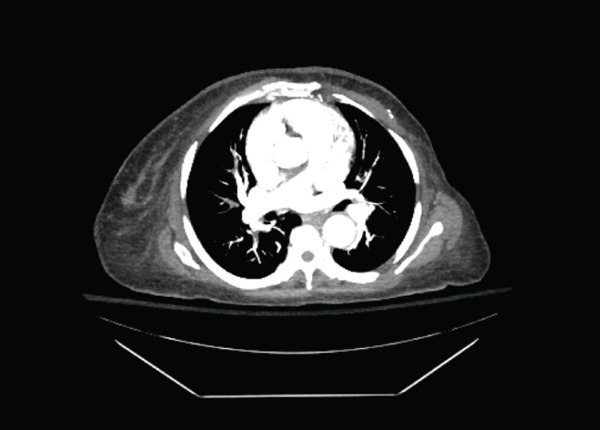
CTA confirming bilateral PE.
